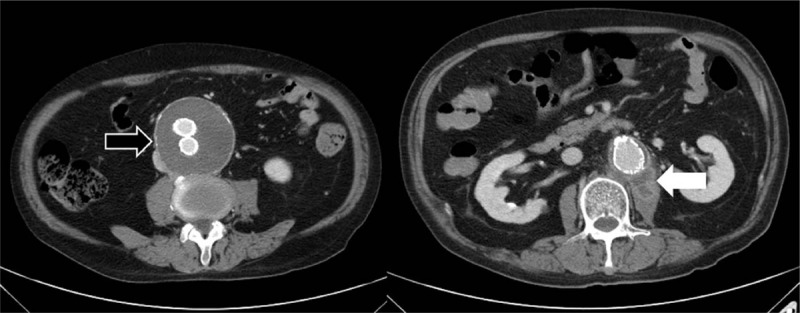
Computed tomography demonstrated a resolution of an endoleak (black arrow) and an irregular enhancement (white arrow) within left psoas muscle about 3 × 1.5 cm in size, connecting with contiguous thromboses aneurysmal sac, which was suggestive of left psoas abscess associated with an infected endograft.
